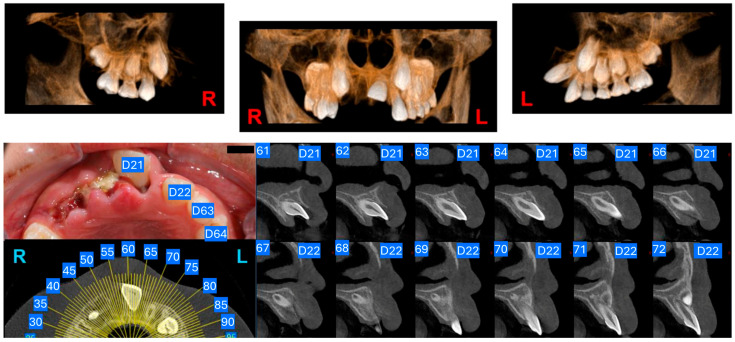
Post-traumatic CT scan showing vestibular luxation with intrusion of tooth 21 and fracture of the buccal alveolar bone, April 2019.
